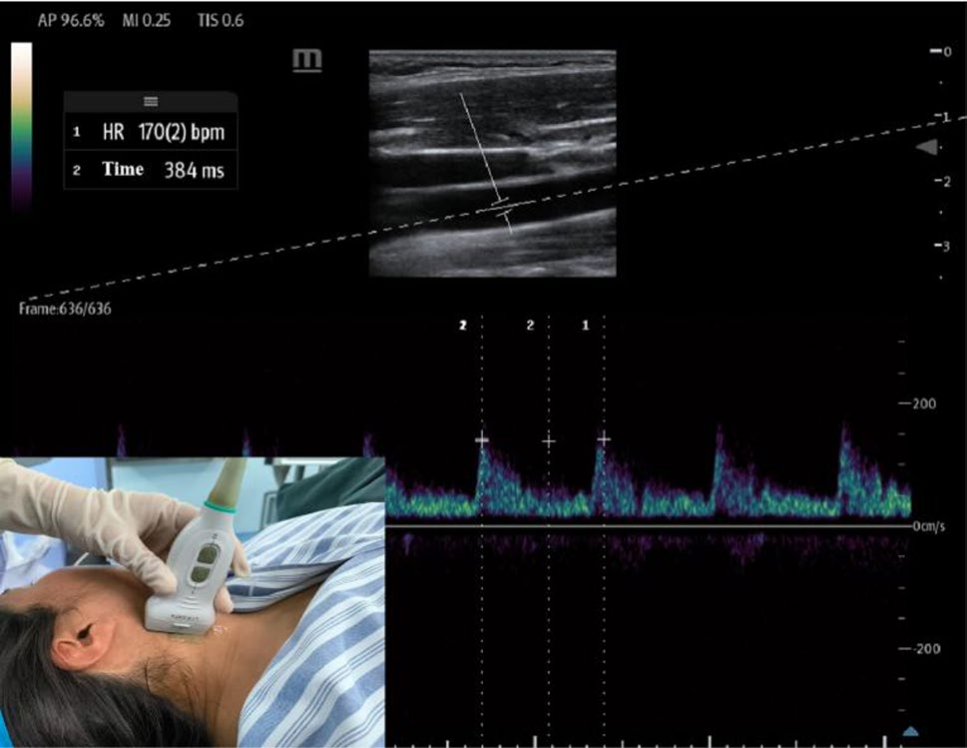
Ultrasound measurement of the blood flow time of the common carotid artery. HR: Heart rate*2; Time: Central blood flow time of the common carotid artery
